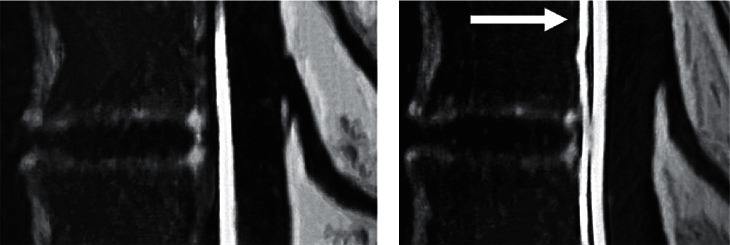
Sagittal view of CT myelography of thoracic spine T10-T11 at 10 seconds (a) and 20 seconds (b) revealing a paravertebral collection indicating a CFS leak (white arrow).
